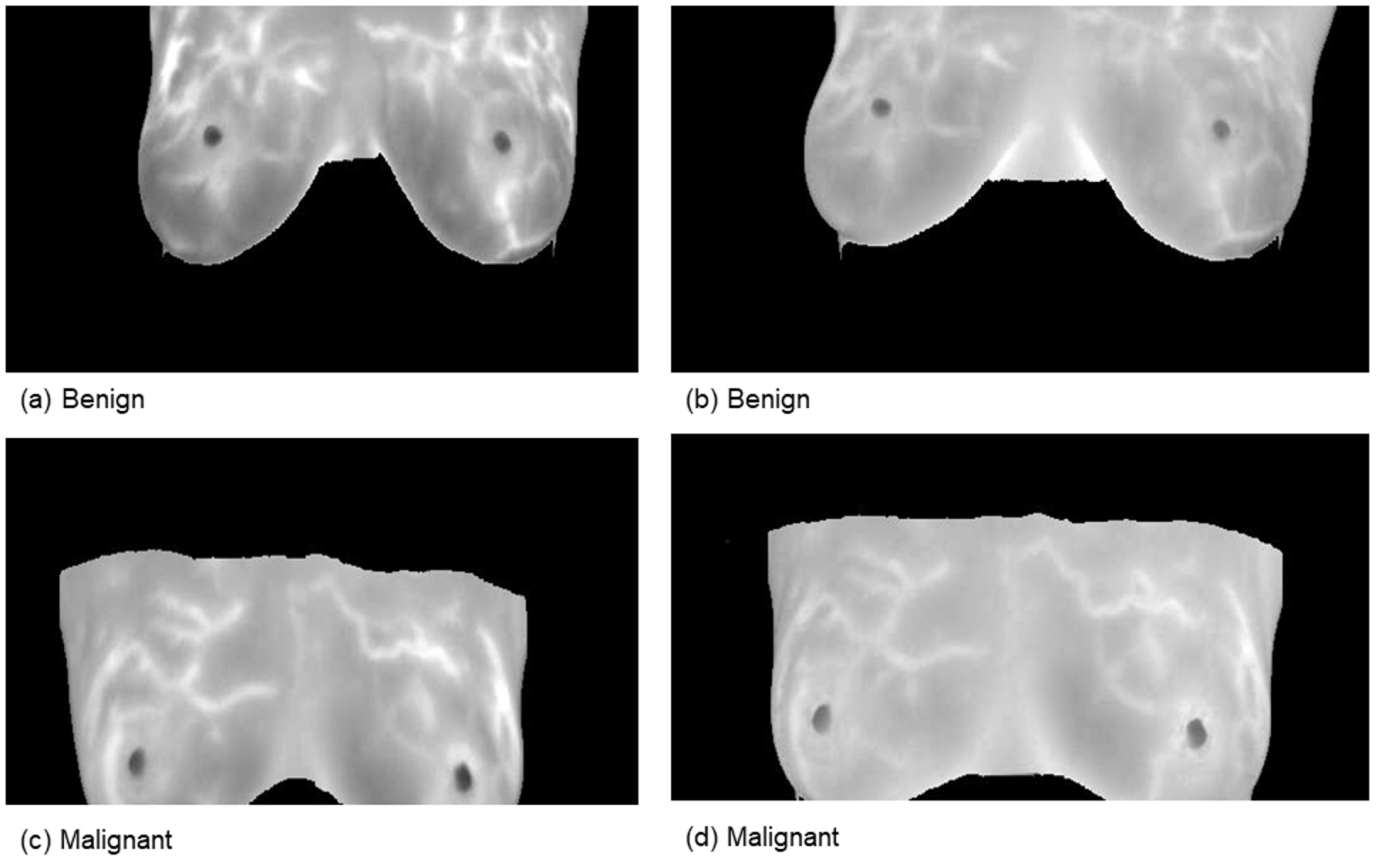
Samples from the magnetic resonance imaging (MRI) dataset.
